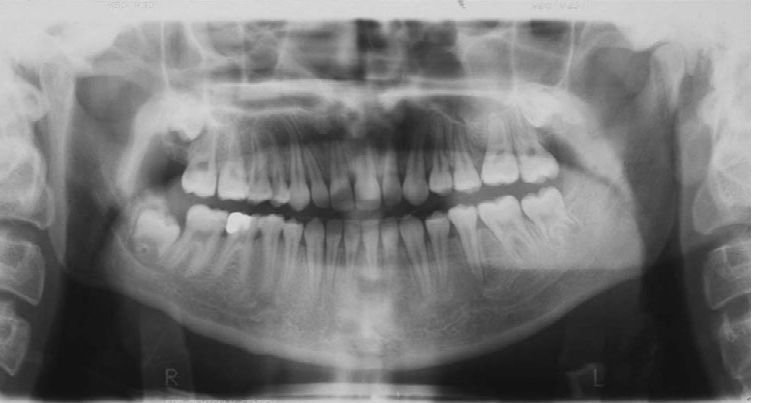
Posttreatment panoramic radiograph of the patient.
